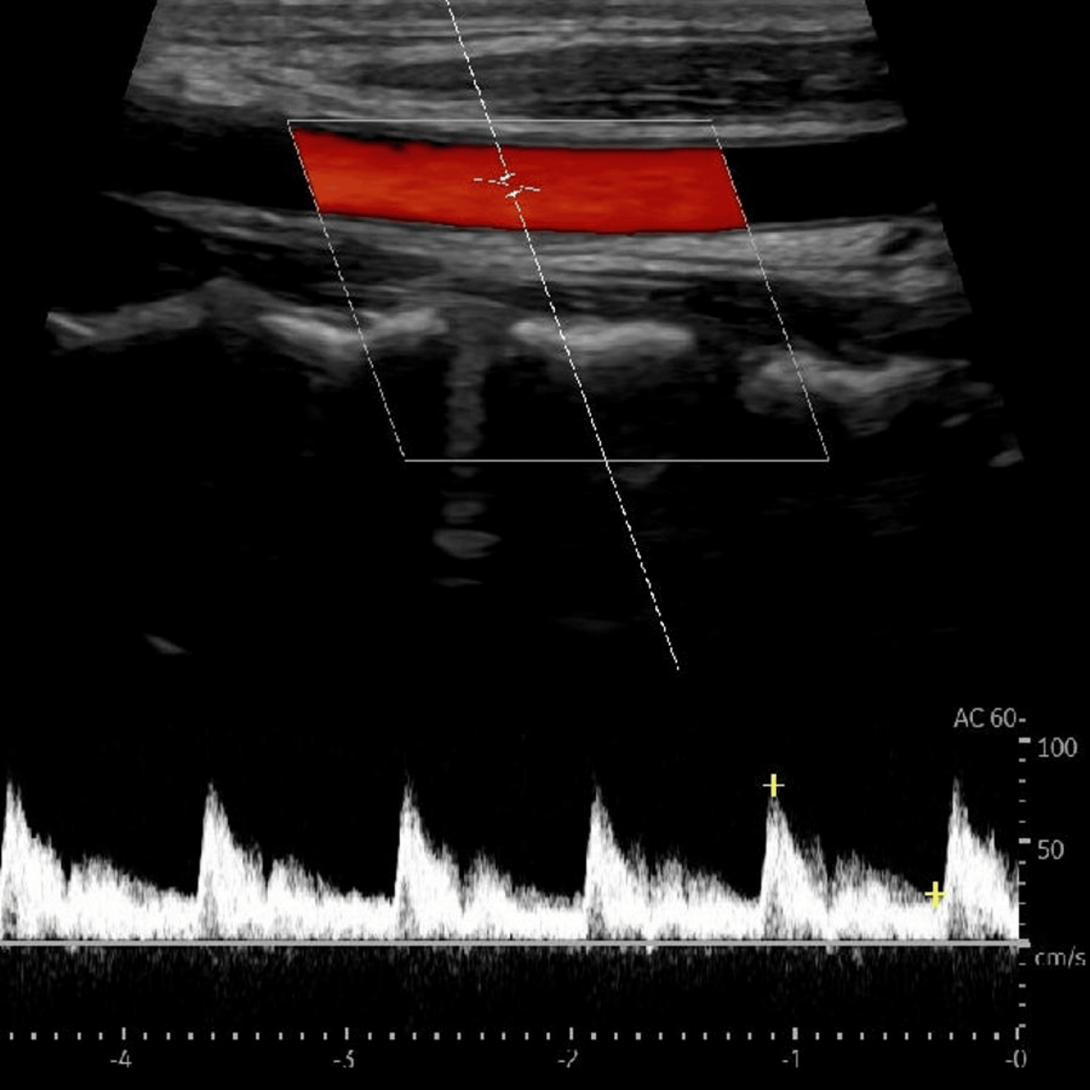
Arterial ultrasound duplex showing a patent vertebral artery stent
